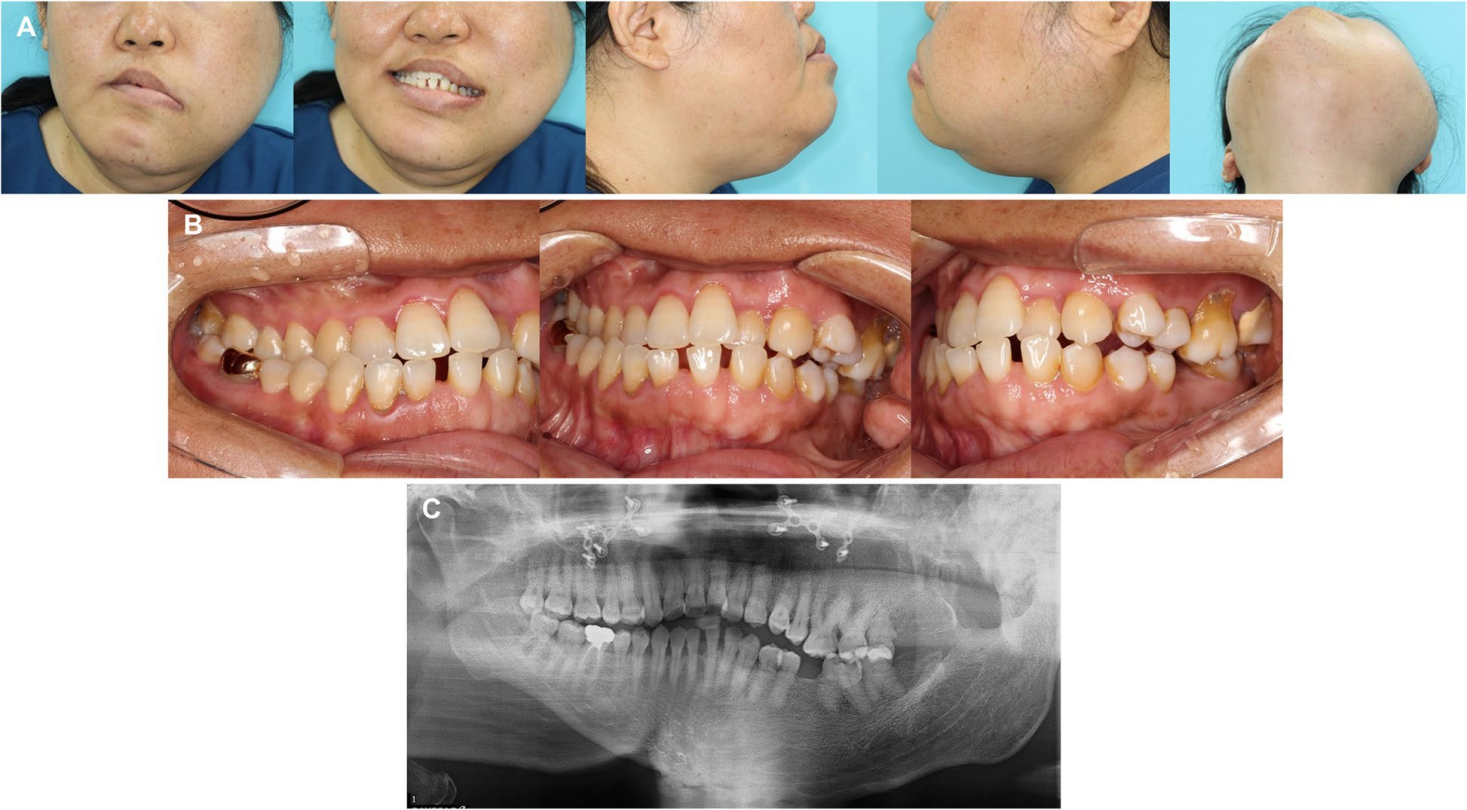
Pretreatment records. A Extraoral photographs. Hypertrophy of the left facial region is observed, accompanied by menton deviation to the right side and facial asymmetry with occlusal canting. B Intraoral photographs. Crossbite and Class III molar relationship are observed on the left side, while scissor bite in the premolar region and Class II molar relationship are observed on the right side. C Panoramic radiographs. Hypertrophy of the left mandible, including the condylar process and coronoid process, is observed, as well as macrodontia in the left posterior region
